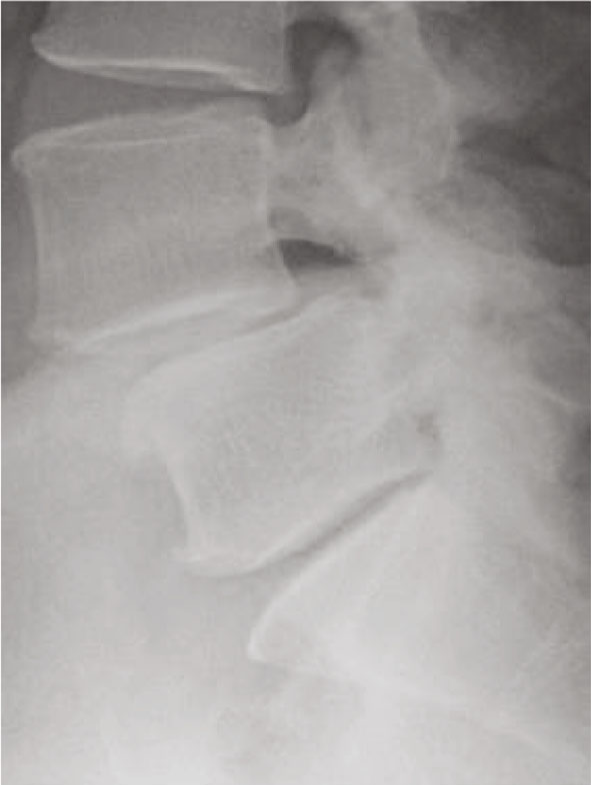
Preoperative lateral lumbar X-ray.
